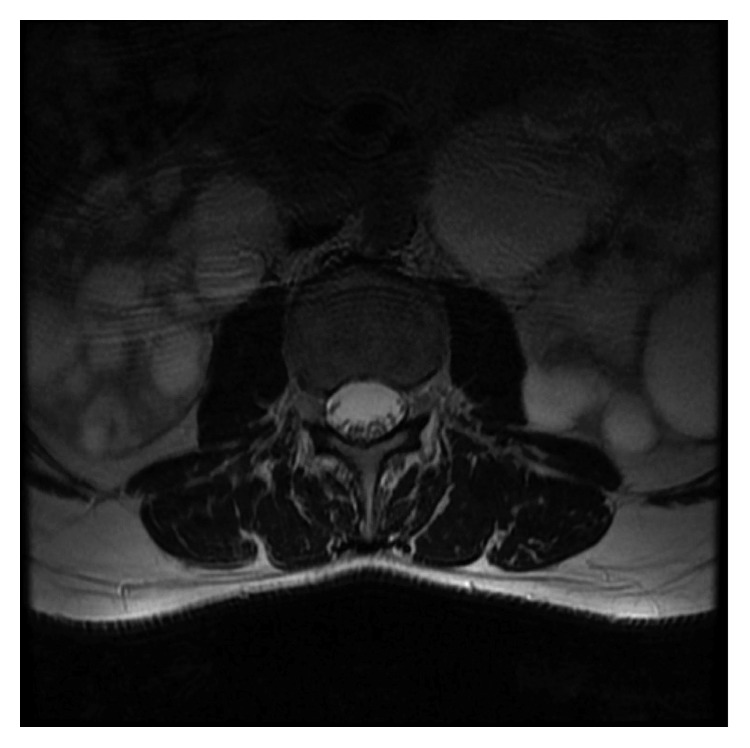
T2-weighted axial MRI scan indicating enlarged kidneys and multiple cysts.
